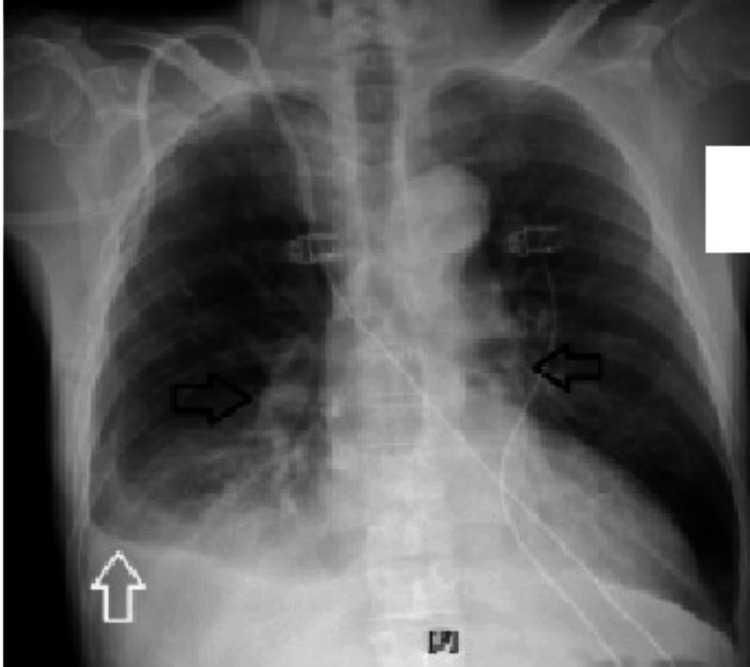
Chest X-ray showing pulmonary congestion (black arrows) and pleural effusion (white arrow).
